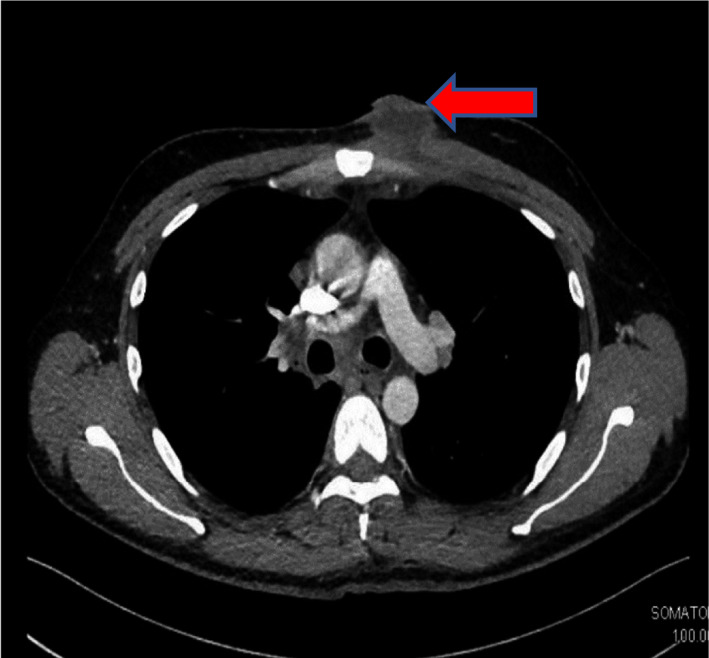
CT chest demonstrating left anterior chest wall abscess with thickened walls (arrow) and clear lung fields
